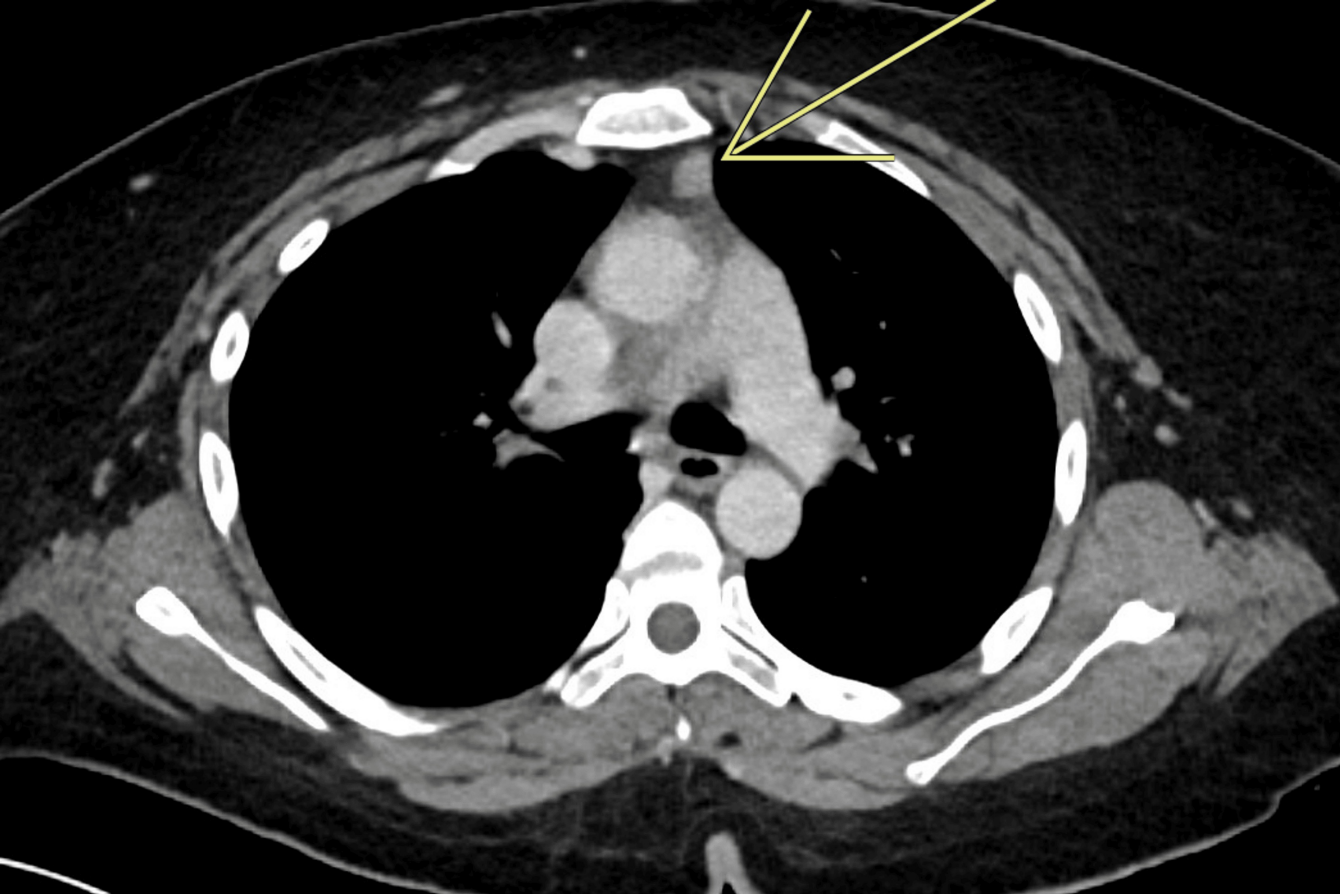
Chest computed tomography (CT) scan showed a small thymic hyperplasia (thymoma type B2, Masaoka-Koga stage 1)
